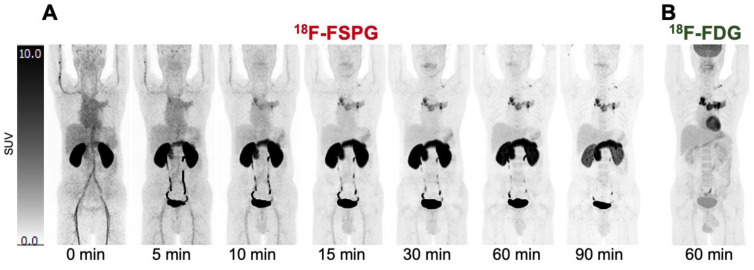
^18^F-FSPG vs. ^18^F-FDG imaging. ^18^F-FSPG scans (SUV range: 0–10) in a patient with metastatic NSCLC at intervals up to 90 min p.i. (A), demonstrating rapid accumulation in the primary and mediastinal metastatic deposits, comparable to a standard 60 min p.i. ^18^F-FDG PET scan (B). The Figure was created from data acquired by Baek S. et al. [37] and provided by Life Molecular Imaging.
